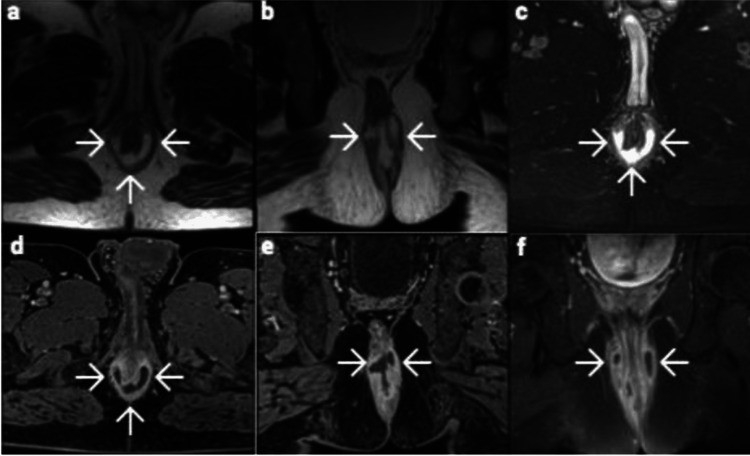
Third patient presenting with a perianal horseshoe fistula and an abscess. Magnetic resonance imaging findings: (a) Axial T2-weighted image (b) Coronal T2-weighted image (c) Axial fat-suppressed T2-weighted image (d) Contrast-enhanced fat-suppressed T1-weighted axial image (e) Contrast-enhanced fat-suppressed T1-weighted coronal image showing rim enhancement (f) Fat-suppressed T1-weighted coronal image
